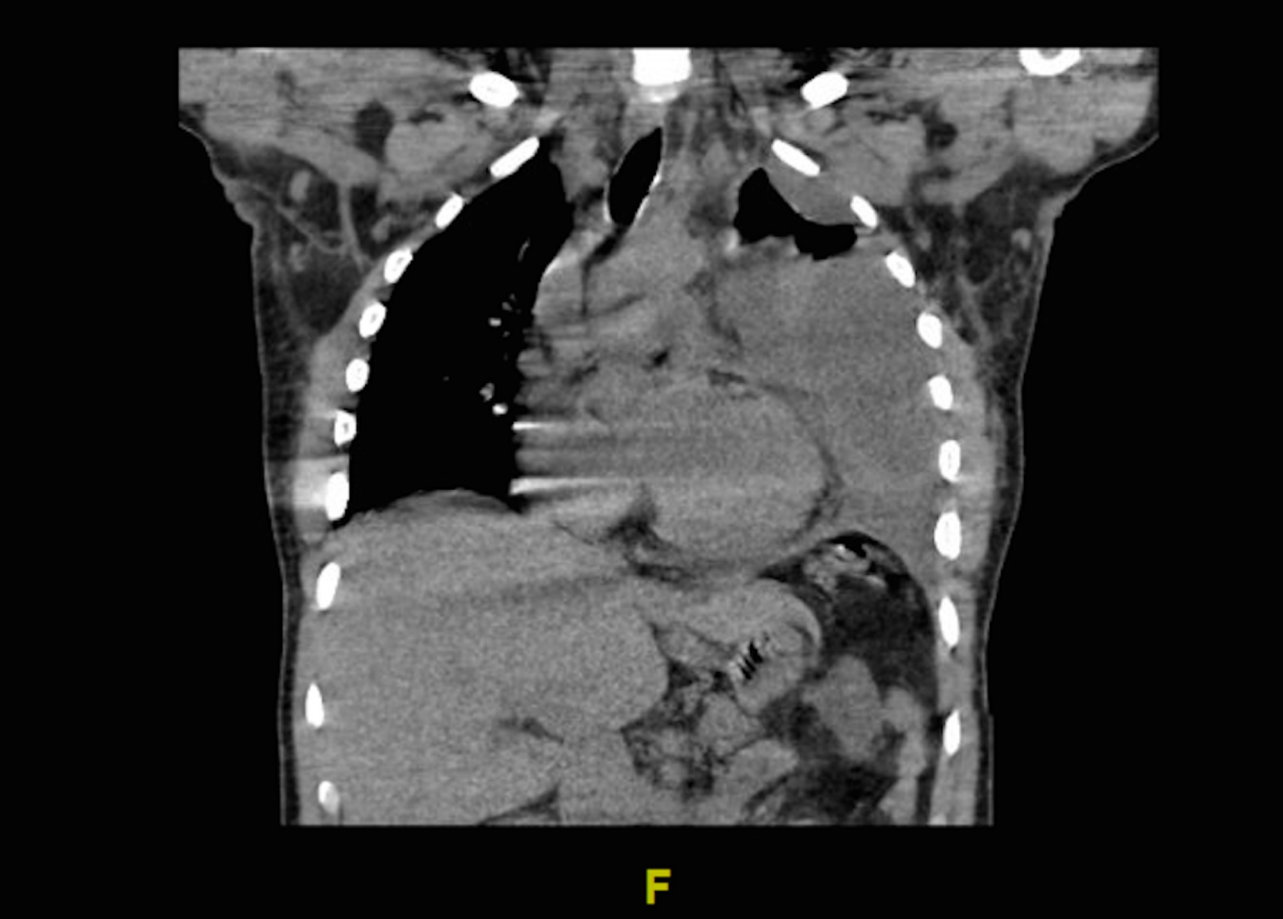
CT of the chest coronal view demonstrating multiloculated left-sided pleural effusion, which developed after IR drainage of the spleen, likely secondary to the splenic abscess or infective endocarditis IR, interventional radiology
